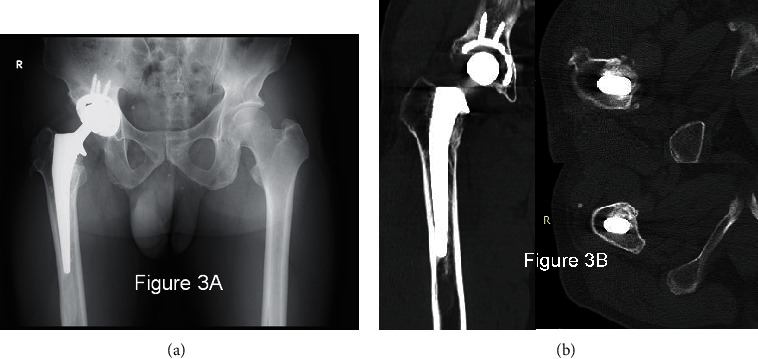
(a) 2-year postoperative X-ray shows minute subsidence and homogenized allograft on the calcar. (b) CT at 2 years proves rigid fixation in Gruen zones 1, 2, and ongoing osteogeneration in other zones.
